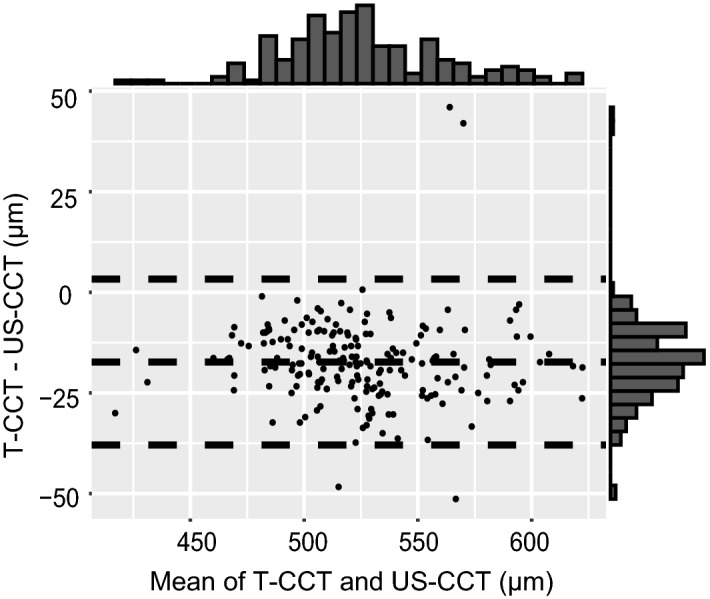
Bland–Altman plots between the CCT measurements of the Topcon noncontact tonopachymeter (T-CCT) and the US pachymeter (US-CCT). The dashed line in the center indicates the mean difference between the two devices. The upper and lower dashed lines are the 95% limits of agreement (LoA). The mean bias was -17.3 µm (95% CI [-18.8, -15.8]; 95% LoA [-37.9, 3.30 µm]). CCT = central corneal thickness; US = ultrasound; CI = confidence interval.
